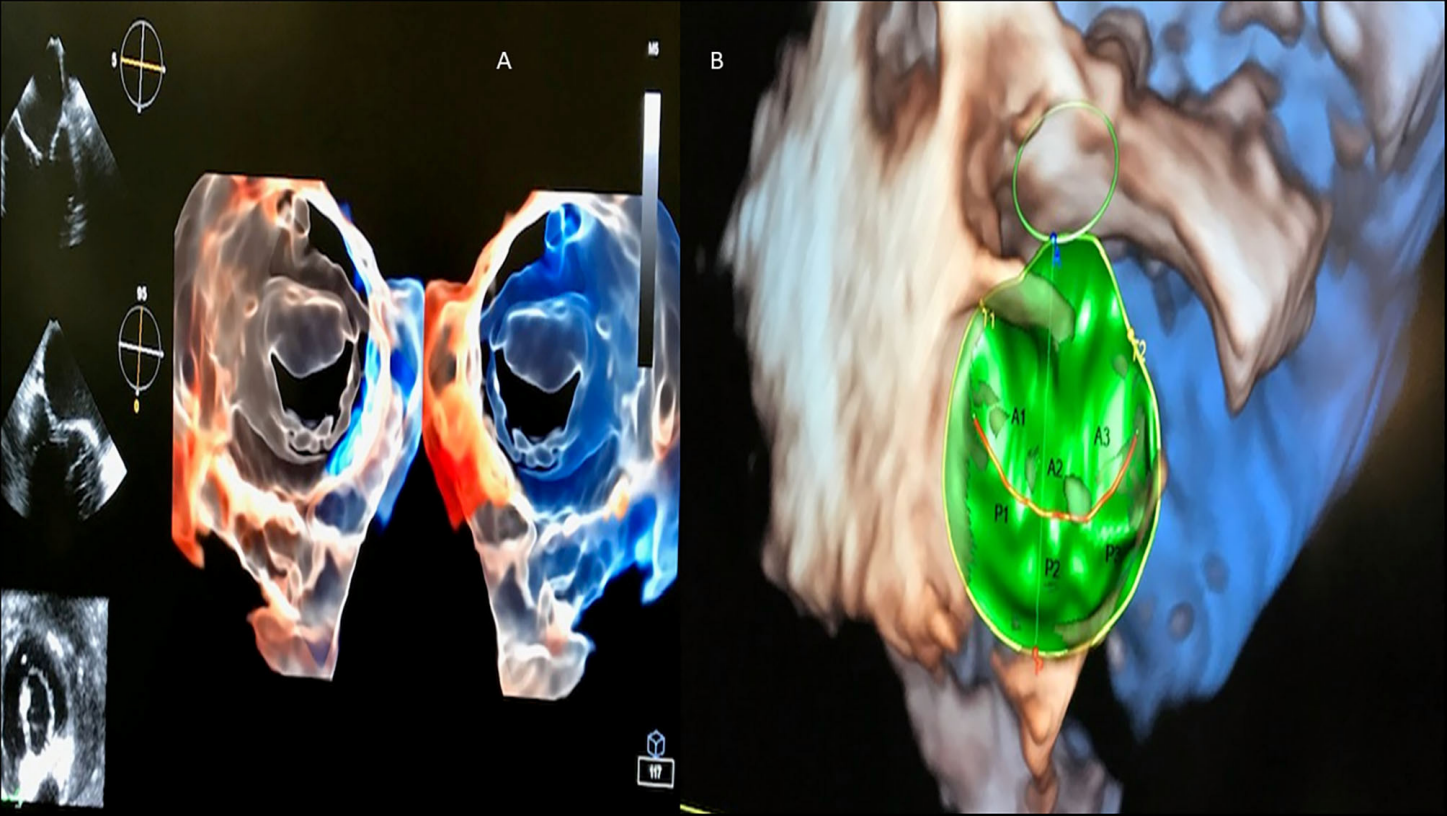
3D transesophageal echocardiography automatic acquisition (in green). (A) Mitral valve (transluminescence technique from atrial and ventricular views); (B) Mitral valve (surgeon view), depicting valvular segments (A1, A2, A3, P1, P2, P3) from anterior (A) and posterior cusps (B).
